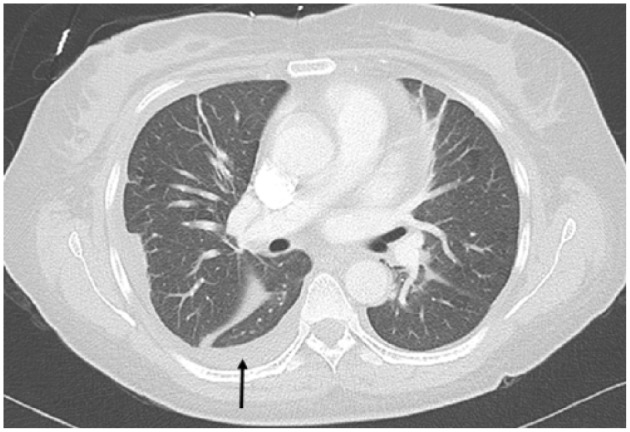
Computed tomography angiography 4 months prior to current presentation. Black arrow showing trace pleural effusion.
